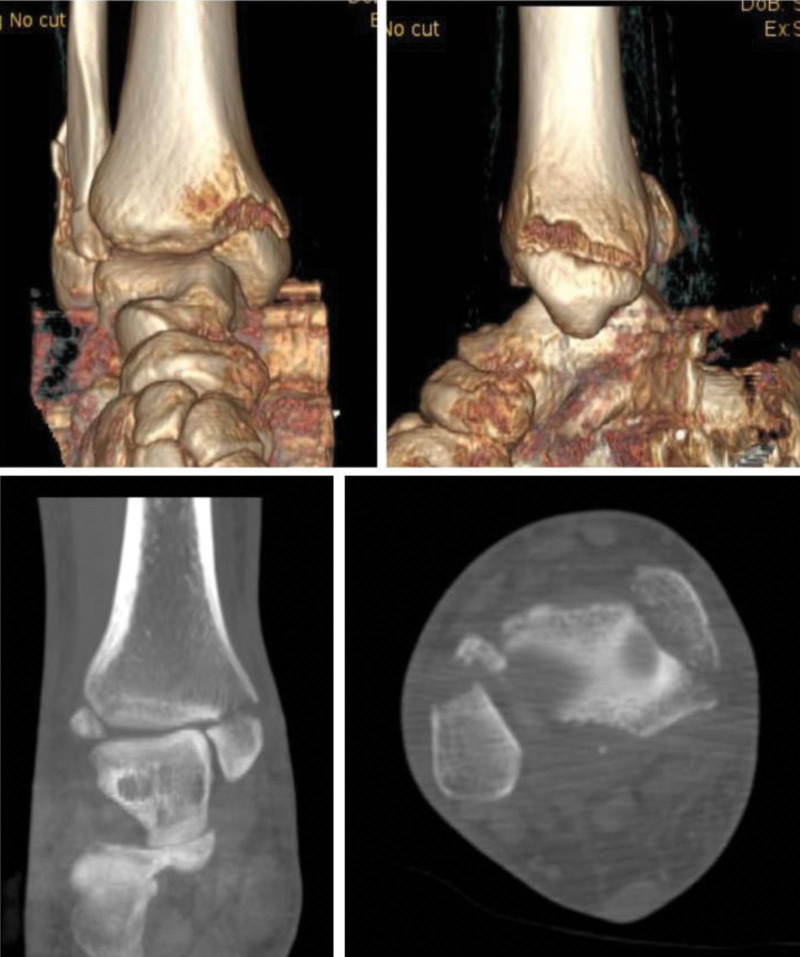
CT imaging after admission. It displayed that the fracture fragment of medial malleolus was large.
